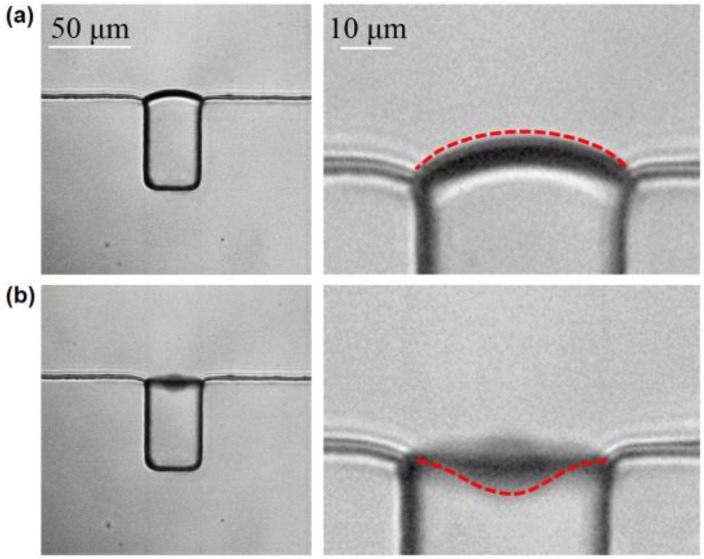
The membrane deformation of the oscillating bubble. (a) No deformation of the oscillating microbubble is observed without ultrasound irradiation. (b) The deformation of the bubble membrane is approximately 16.23 μm when the input voltage is 144 Vpp.
